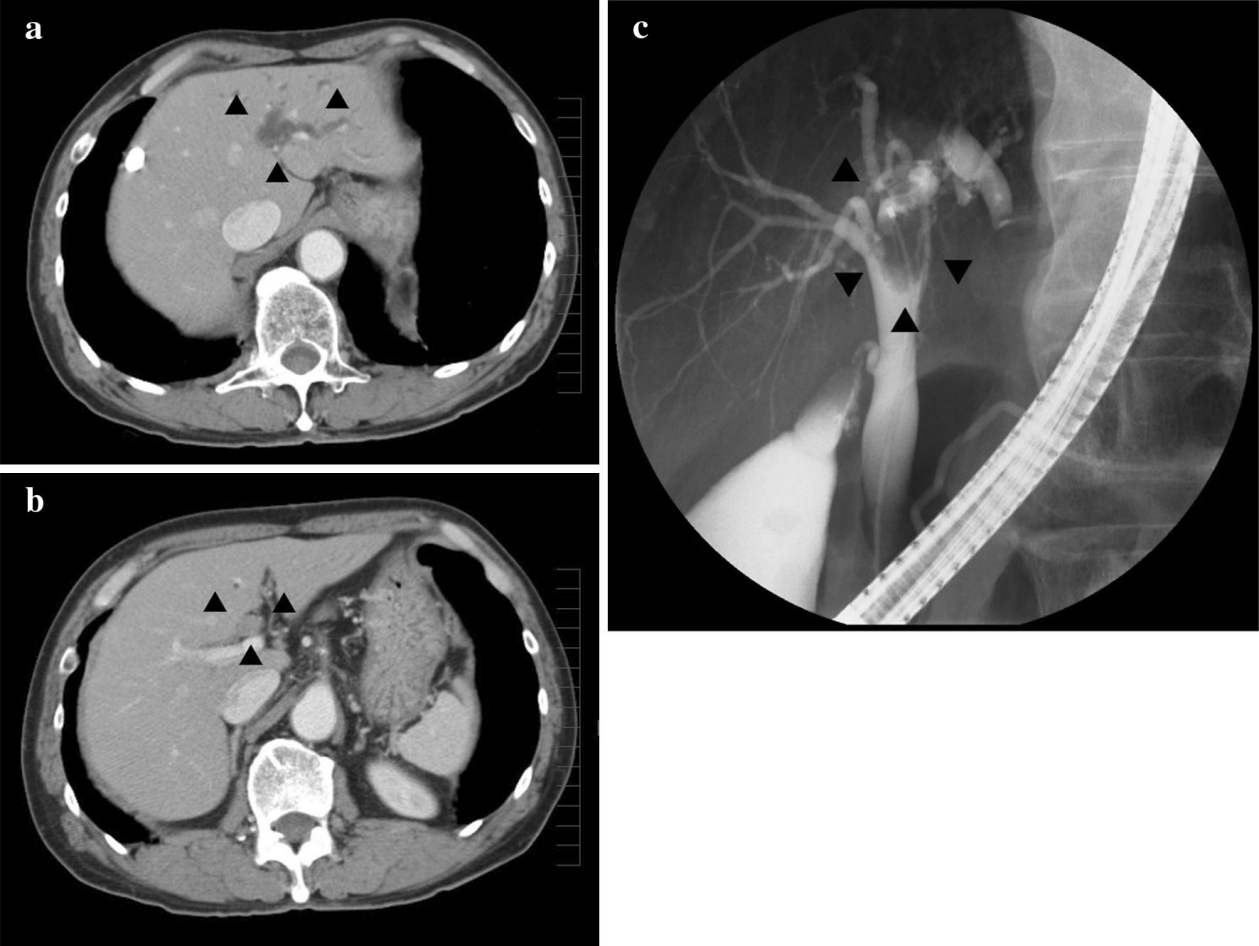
Imaging of the hepatobiliary system. Computed tomography (a, b) and endoscopic retrograde cholangiography (c) revealed a mass in the left hepatic bile duct and a dilated left intrahepatic bile duct (*arrowheads*)
